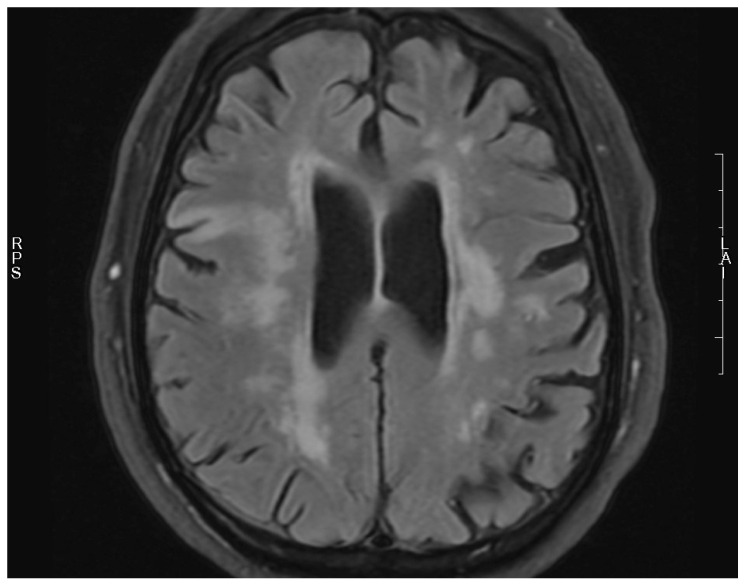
Chronic infarct and its volume on FLAIR sequence—106,593 cm^3^ (and stenosis location (internal carotid artery cavernous segment, “RPS” right-posterior-superior, “LA” left-anterior).
